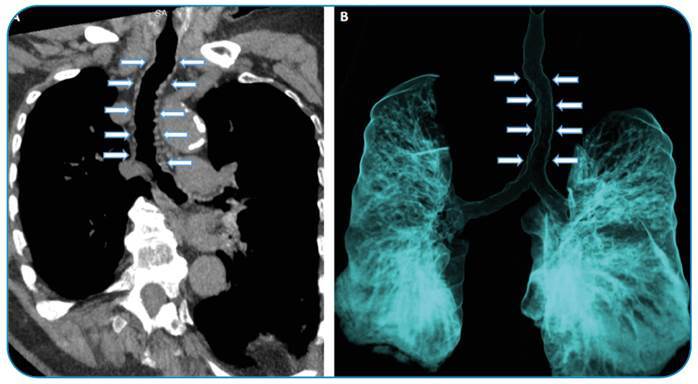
Chest computed tomography findings in the coronal (A) and air-specific reformat (B) views demonstrating diffuse tracheal papillomatosis (arrows).
